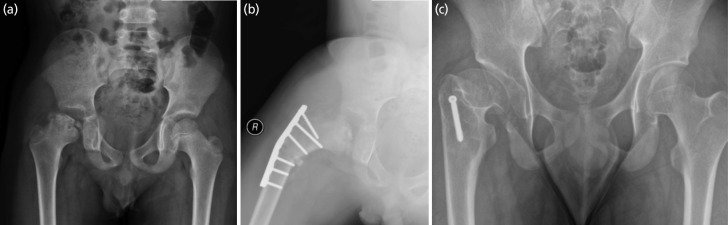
The series of radiographs belongs to patient no.3. (a) The presentation at the age of 9 showed fragmentation phase with Herring C. (b) The immediate post-surgery showed good containment. (c) The hip at the age of 16 years old showed Stulberg III. Overgrowth of the greater trochanter with the presence of a screw indicates failure of trochanter epiphysiodesis done at the age of 10 years old.
